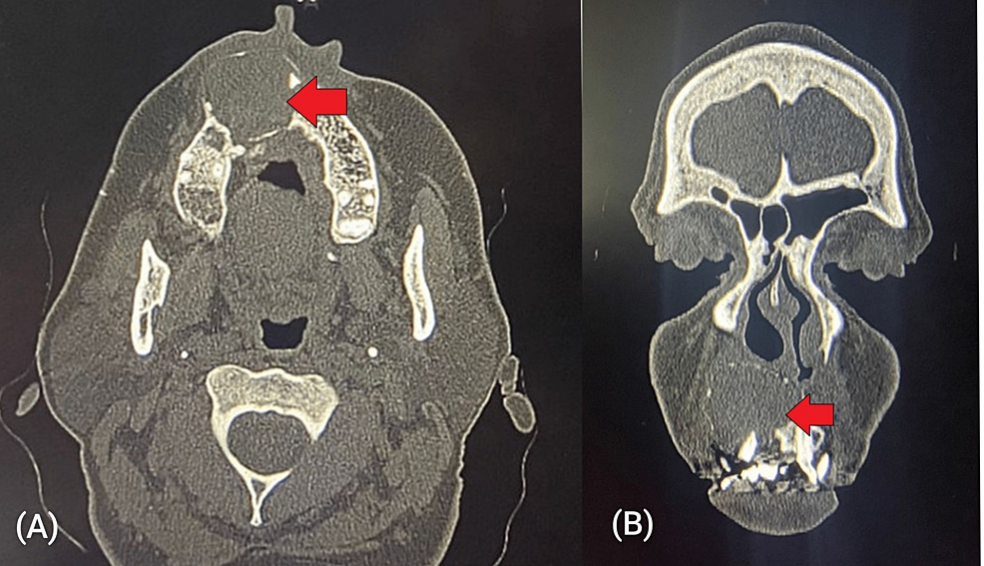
(A) CT image (axial section) of mucoepidermoid carcinoma of the hard palate; (B) CT image (coronal section) of mucoepidermoid carcinoma of the hard palate CT: computed tomography
